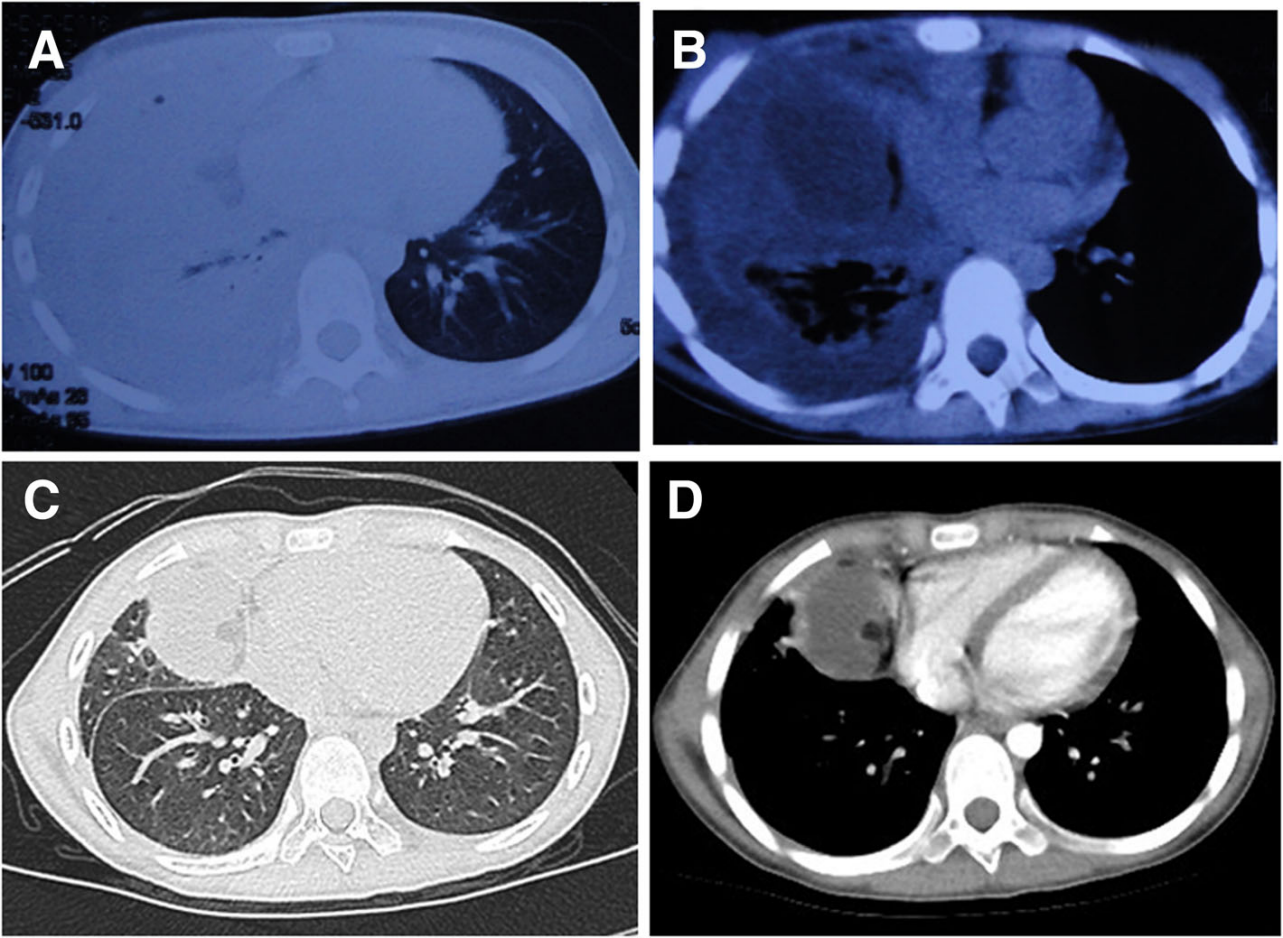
Lung CT showing the presence of 3A right-sided pulmonary consolidation and massive pleural effusion, 3B pachypleuritis and low density mass, and 3C-D a right-sided mass with multiple focal fatty densities adjacent to the heart
